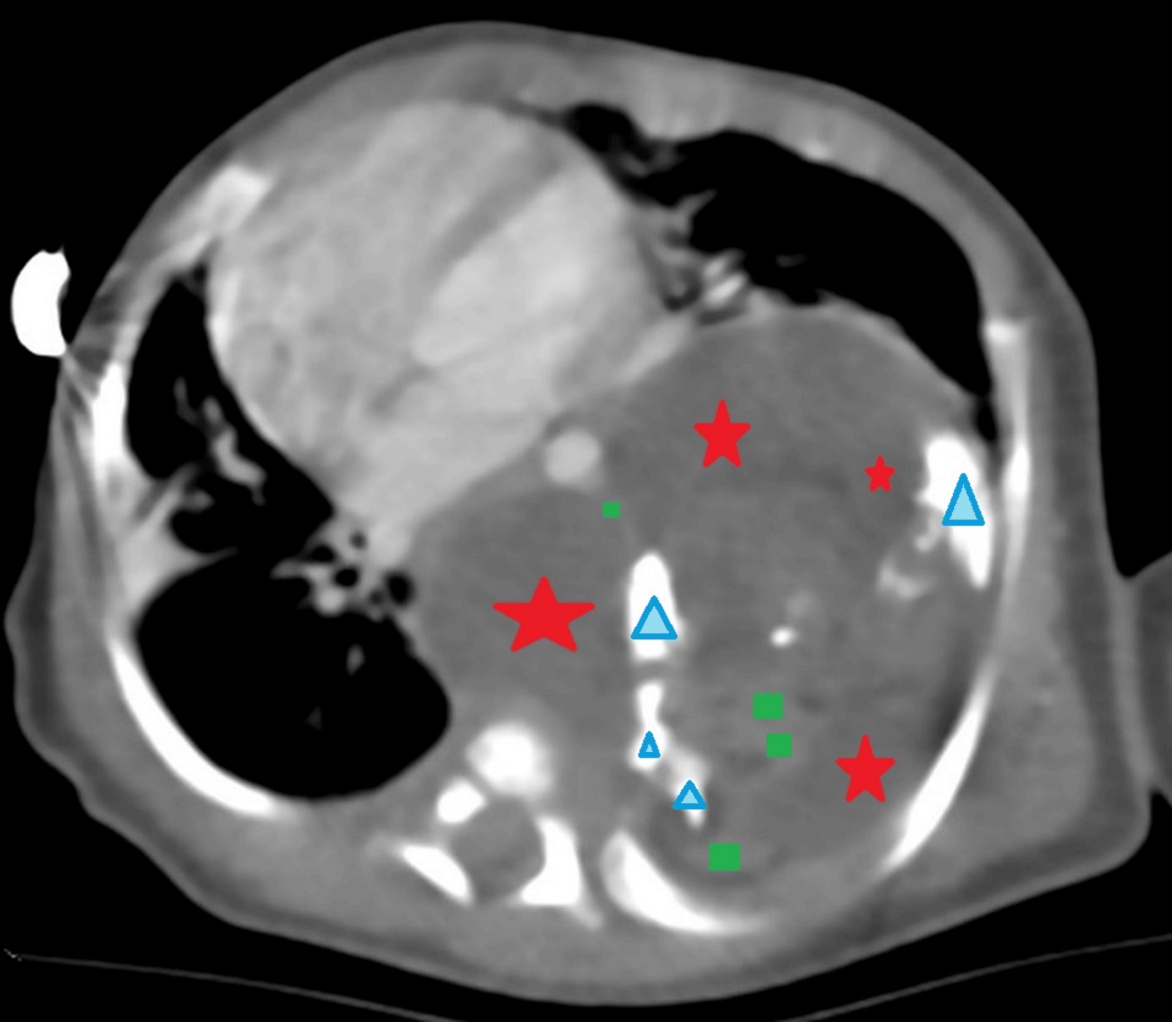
Injected thoracic CT scan objectifying the teratoma (axial section) Injected thoracic CT scan at arterial phase in the mediastinal window demonstrating a voluminous mass with three components: cystic (red marks), tissue (green mark), and calcified (blue marks), associated with deviation of mediastinal structures toward the contralateral side.
